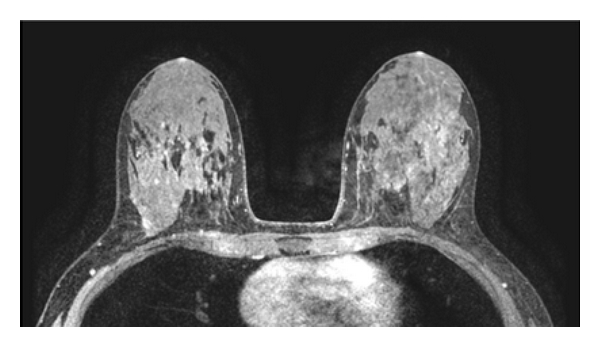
MIP image from breast MRI showing multiple regions of enhancement with no mass in the area of clinical concern left breast.
